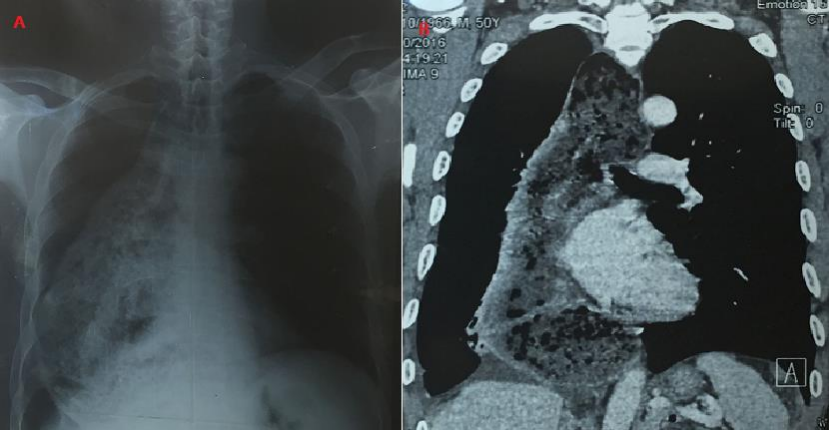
(A) radiographie thoracique de face: opacité inhomogène occupant les lobes moyen et inférieur du poumon droit; (B) scanner thoracique: méga-œsophage thoracique mesurant 78 mm de diamètre associée à une pleuropneumopathie droite
